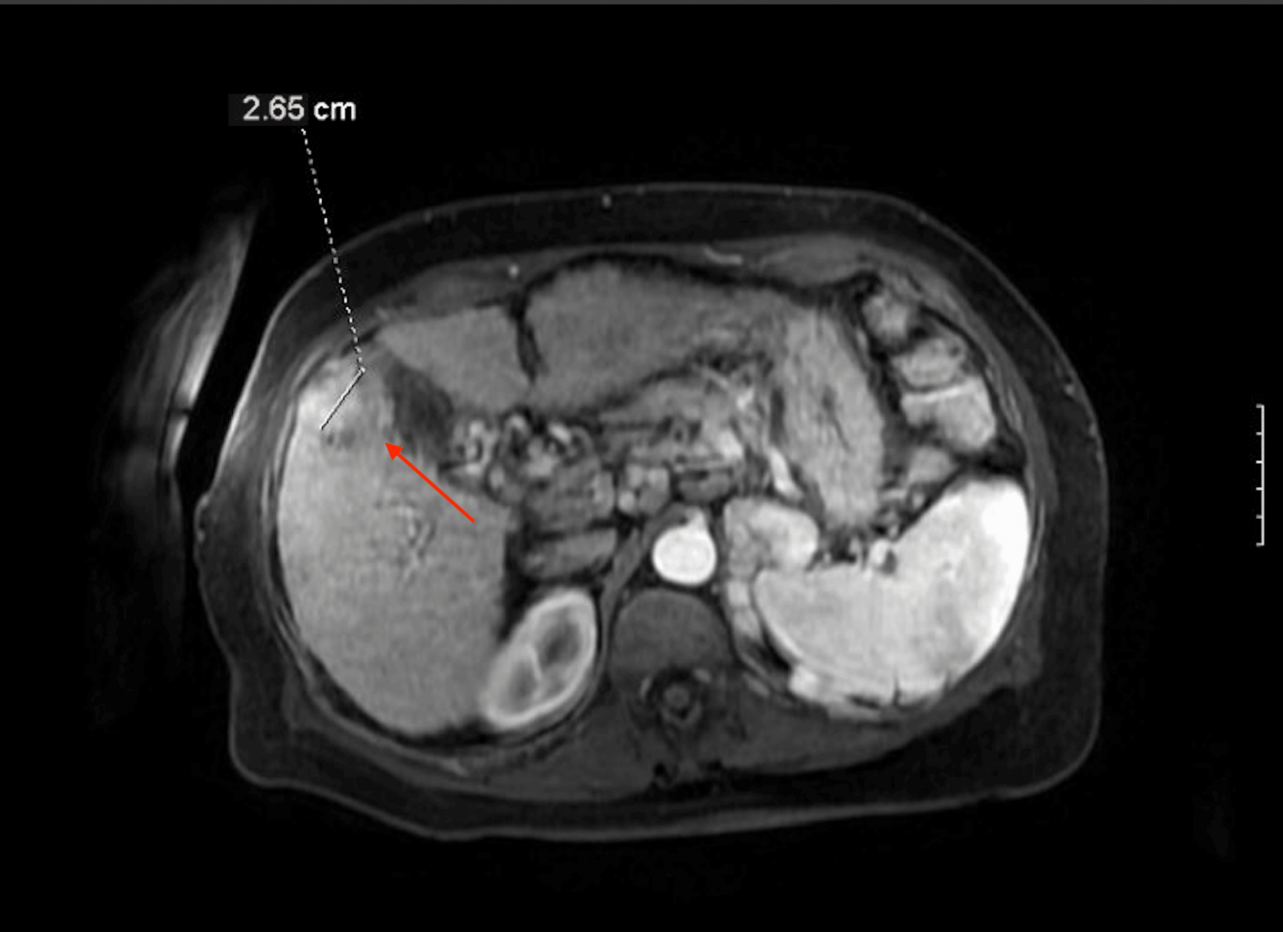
MRI of the abdomen with and without contrast characterizing HCC (arrow) as LI-RADS 5 in segments VIII and V MRI: magnetic resonance imaging, HCC: hepatocellular carcinoma, LI-RADS: Liver Imaging Reporting and Data System
